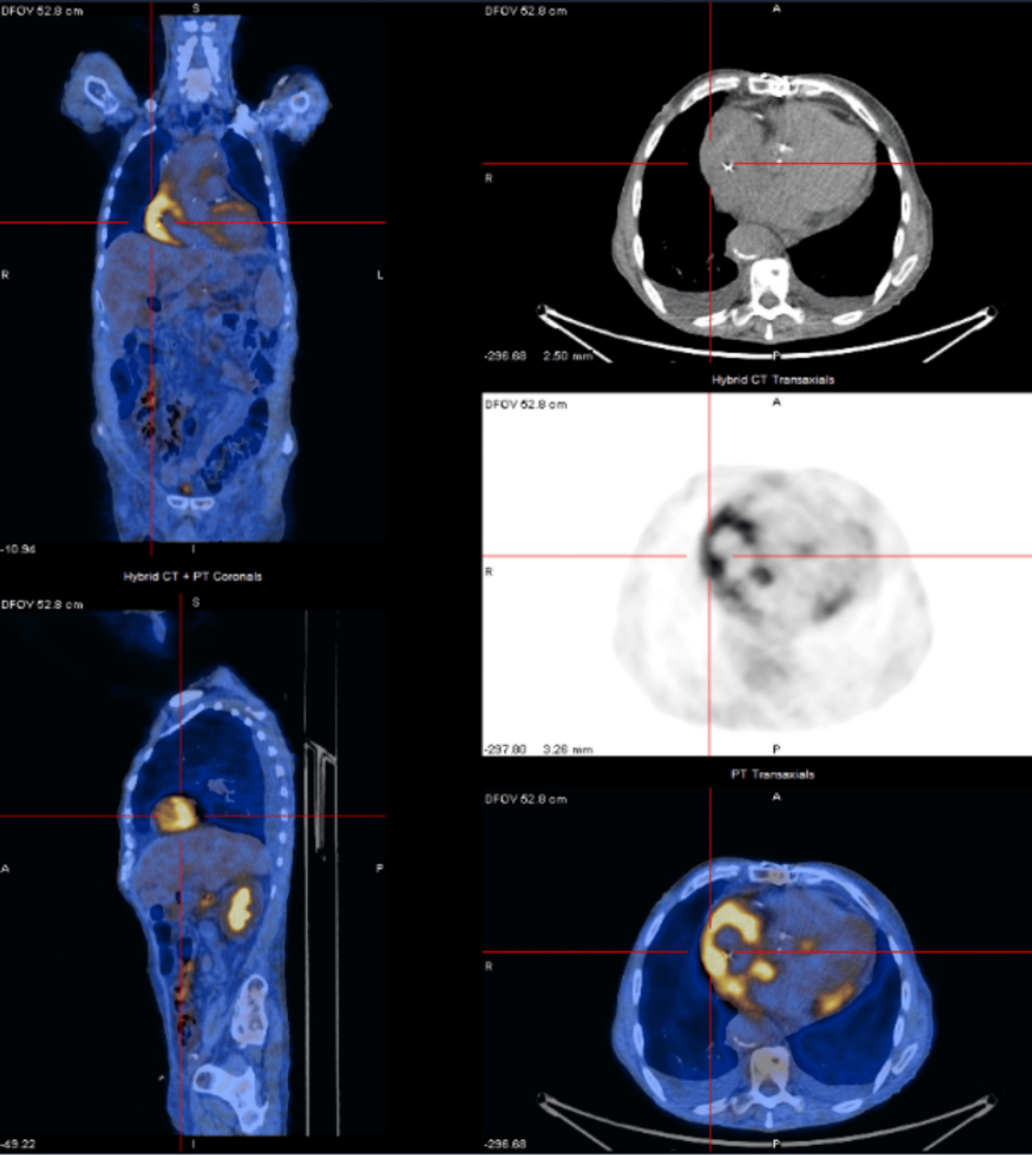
Whole body 18F-FDG PET-CT revealing increased FDG uptake in the myocardium of the right atrium, affected by the disease FDG PET-CT: fluorodeoxyglucose positron emission tomography-computed tomography
